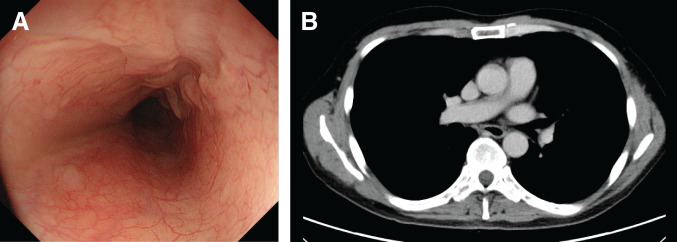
Upper gastrointestinal endoscopy and contrast-enhanced CT after FP plus pembrolizumab. (A) The tumor lesion had flattened and was covered with normal mucosa. (B) The tumor and enlarged lymph nodes had reduced in size. FP, 5-fluorouracil and cisplatin
